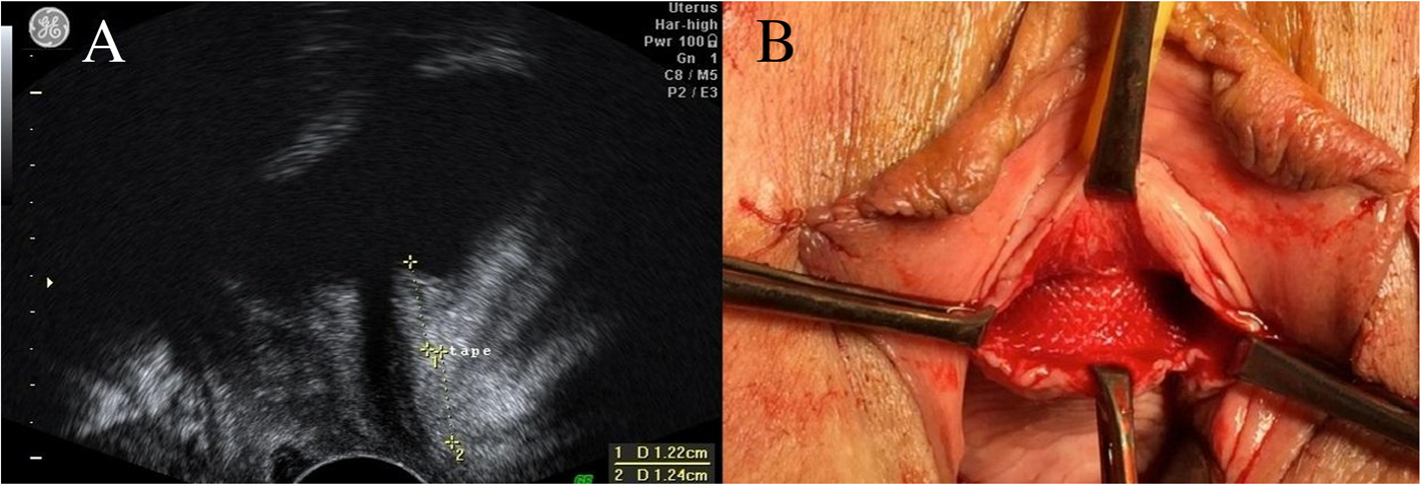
a Ultrasound evaluation of the tape after surgery: D1 distance from bladder neck to the middle of the tape, D2 distance from the middle of the tape to the external urethral meatus. b Tape under the urethra
